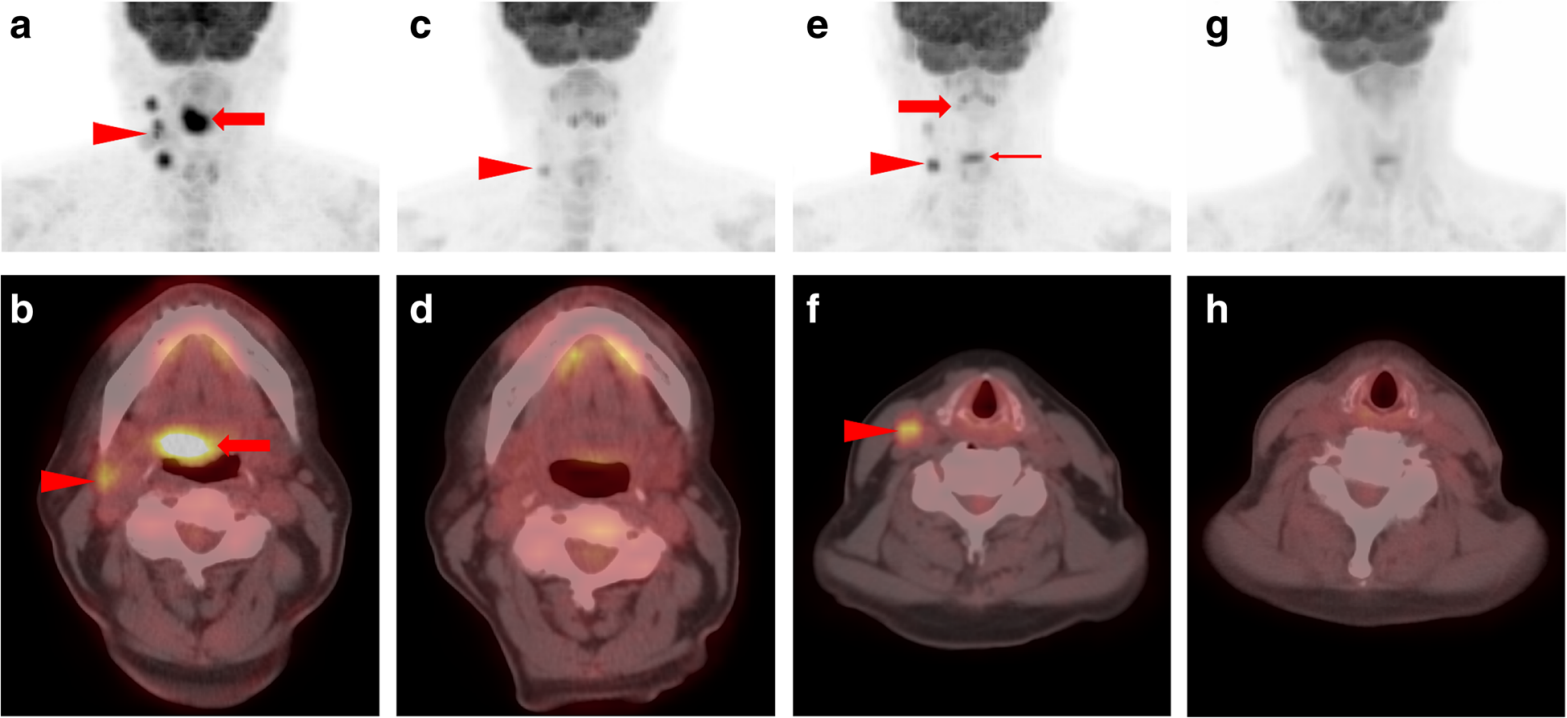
a Baseline head and neck maximum intensity projection (MIP) image demonstrating focal FDG uptake in the patient’s BOT HNSCC (red arrow) as well as ipsilateral cervical adenopathy (red arrowhead). b Representative axial PET/CT slice from the same time point as in (a) which delineates the BOT HNSCC (red arrow) and also highlights one of the right-sided cervical lymph nodes (red arrowhead). c Head and neck MIP image following 3 cycles of R-CHOP demonstrates complete metabolic response in the patient’s BOT HNSCC and partial response in the ipsilateral cervical adenopathy (red arrowhead). d Axial PET/CT image from the same time point as (c) shows no abnormal uptake at the BOT (persistently FDG-avid cervical nodes are not shown on this slice). e Head and neck MIP image following completion of R-CHOP therapy demonstrates very subtle increased uptake in the BOT HNSCC (red arrow, barely visible) and increasing uptake in ipsilateral cervical lymph nodes (red arrowhead). Note normal physiologic activity in the vocal cords (thin red arrow). f Representative axial PET/CT image through the neck shows an FDG-avid right level III lymph node compatible with residual HNSCC. g Head and neck MIP and (h) axial PET/CT images following completion of chemoradiation therapy show no evidence of metabolically active primary or nodal HNSCC
